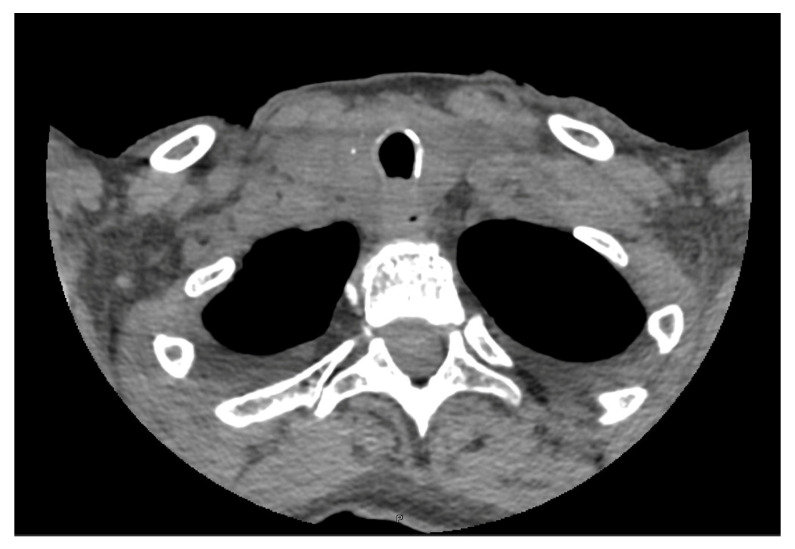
CT of the neck demonstrating narrowing of the cervical portion of the esophagus by TM.
